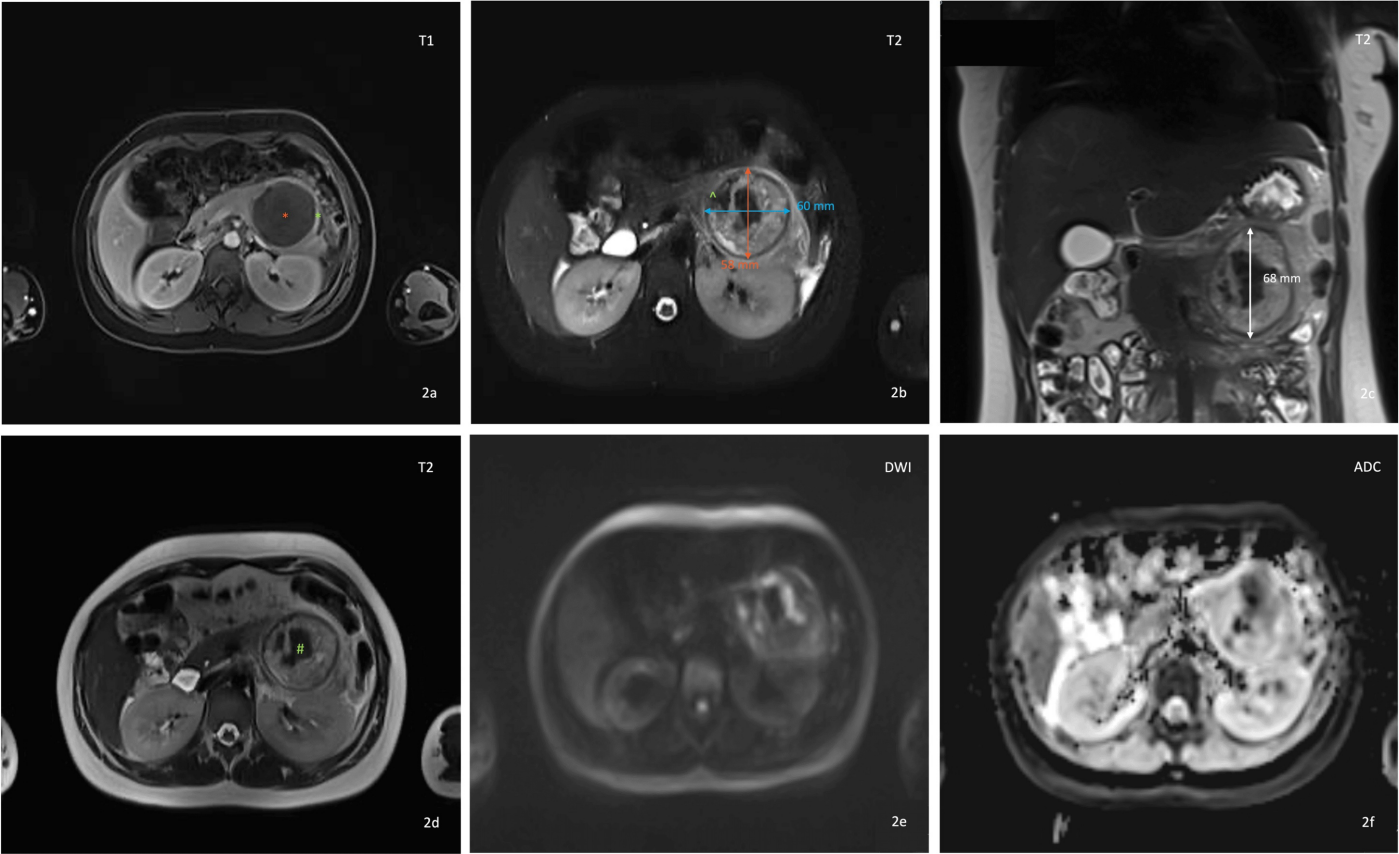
Abdominal magnetic resonance imaging (MRI) with cholangiographic study A large heterogeneous mass (red “*”) arising from the pancreatic tail was identified (Figure 2a), measuring approximately 68 × 60 × 58 mm on axial (Figure 2b) and coronal images (Figure 2c). Figure 2a (axial fat-suppressed T1-weighted post-contrast sequence) demonstrated mild peripheral enhancement (green “*”) after intravenous gadolinium administration. Figure 2b (axial fat-suppressed T2-weighted post-contrast sequence) demonstrated disruption of the wall/capsule (green “^”) at the anteromedial aspect, with posterior extension of the inflammatory process, predominantly at the posteroinferior aspect. This finding was associated with edematous infiltration of the adjacent tissue planes and a small fluid collection extending into the left anterior pararenal space and the left paracolic gutter. Figure 2d (axial T2-weighted sequence) showed that the lesion had a visible wall and displayed a heterogeneous T2 signal (green “#”), with areas of hypointensity, intermediate signal, and hyperintensity. Figure 2e and Figure 2f (axial diffusion-weighted magnetic resonance images (DWI) acquired with a b value of 1500 s/mm² and the corresponding apparent diffusion coefficient (ADC) map) showed no true diffusion restriction, as indicated by the absence of signal hyperintensity on DWI and the lack of corresponding signal reduction on the ADC map.
